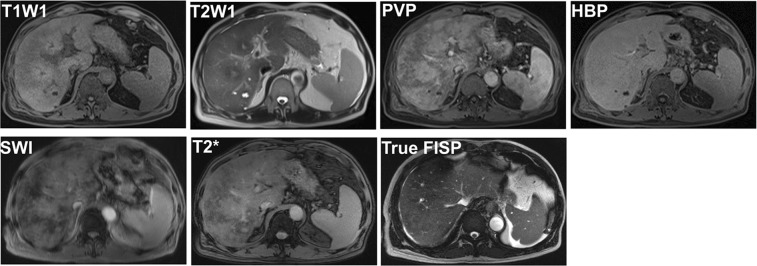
67-year-old man with PAs-induced HSOS received MRI scan, and the images of MRI SWI and T2*WI displayed the lesion more clearly than pre-contrast sequences (T1W1 and T2W1). Similar distribution of hypointensity was observed in SWI/T2*WI and portal-venous phase (PVP) of dynamic contrast-enhanced (DCE) MRI; while, distributional similarity between SWI/T2* imaging and HBP was not observed. PVP: portal-venous phase; HBP: hepatobiliary phase.
